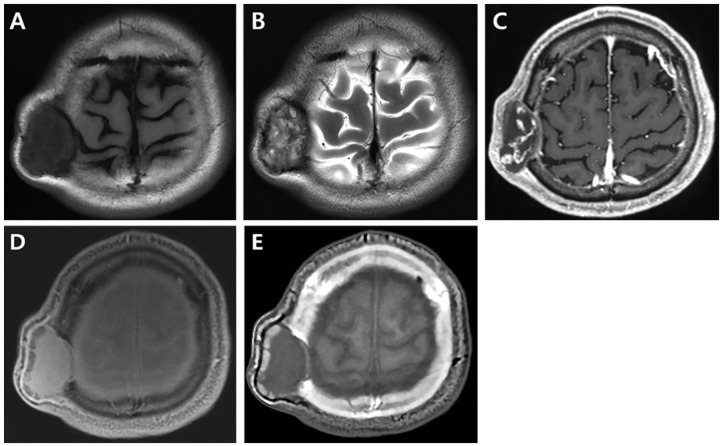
Magnetic resonance imaging of the brain. (A) On axial T1-weighted image, the mass demonstrates inhomogeneous hypointensity with dark inner margin. (B) On axial T2-weighted image, the expansile intraosseous mass shows heterogeneous hyperintensity with hypointense peripheral rim and internal septa. (C) On contrast enhanced axial T1-weighted image, the mass reveals multifocal serpentine enhancing components. There is no combined abnormality in the adjacent brain parenchyma. (D,E) Axial proton-density (D) and computed tomography (CT)-like contrast (E) images from zero echo time (ZTE) sequence showing the mass in the right parietal bone with destruction of inner and outer tables and peripheral bone fragments along the outer margin. Findings of ZTE magnetic resonance imaging are similar to those of the CT image with bone window setting (Figure 2C).
